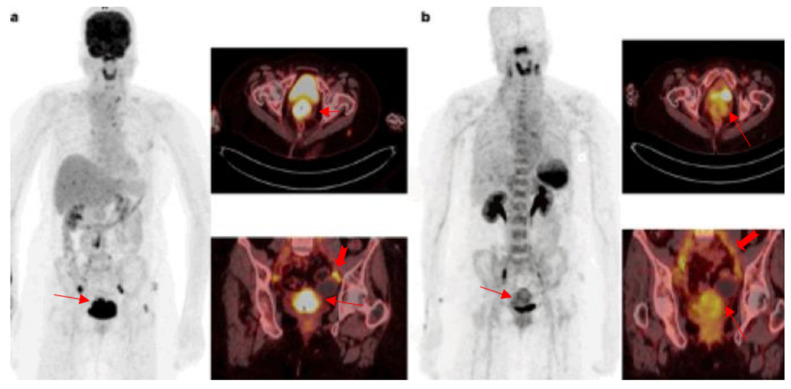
^18^F-FDG and ^68^Ga-Pentixafor images of a 36-year-old female patent with squamous cell carcinoma of the cervix with intense uptake in the primary lesion and pelvic lymph node metastasis on ^18^F-FDG (a). There is moderate heterogenous tracer accumulation on the ^68^Ga-Pentixafor PET and very low uptake on the metastatic lymph node (b).
